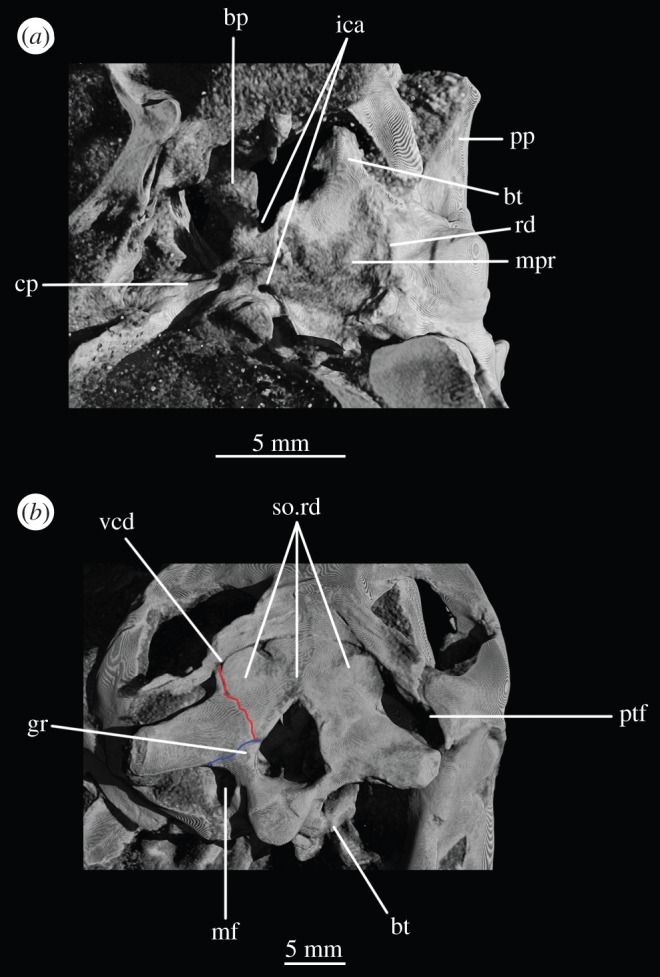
CT reconstructions of braincase of SAM-PK-5867 in (*a*) ventral and (*b*) posterior views. In (*b*), red line indicates the suture between supraoccipital and opisthotic and blue line indicates the suture between opisthotic and exoccipital. For abbreviations, see table 1.
